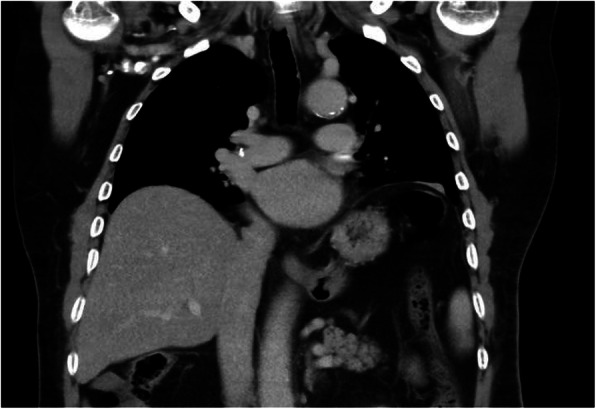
Post-operative chest CT scan, coronal view, mediastinal window showing diaphragmatic elevation
